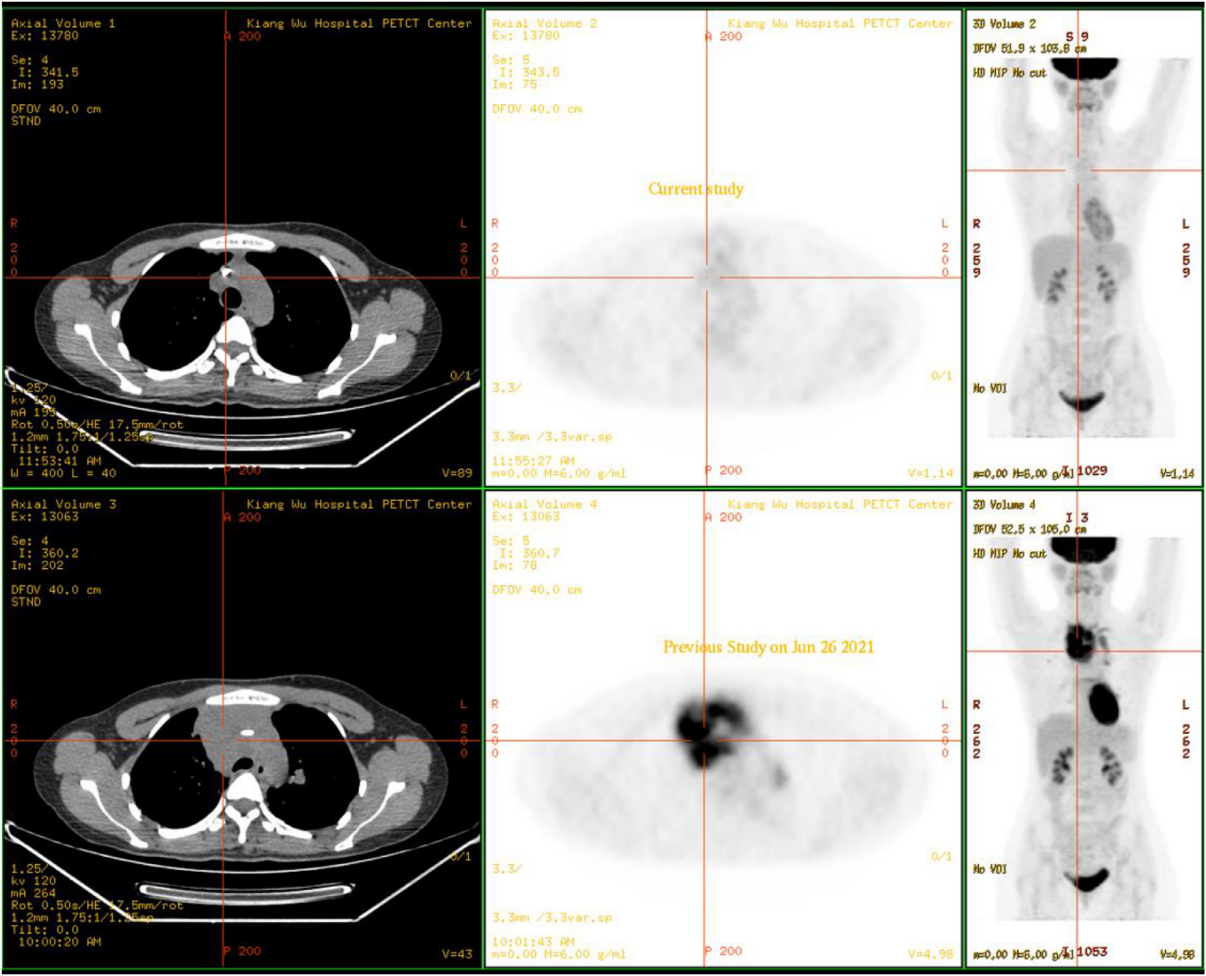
The ^18^F-FDG PET/CT scan showed complete metabolic remission. Post PD-1 inhibitor and targeted therapy, the ^18^F-FDG PET/CT scan on October 19, 2021 showed complete metabolic remission of the disease.
